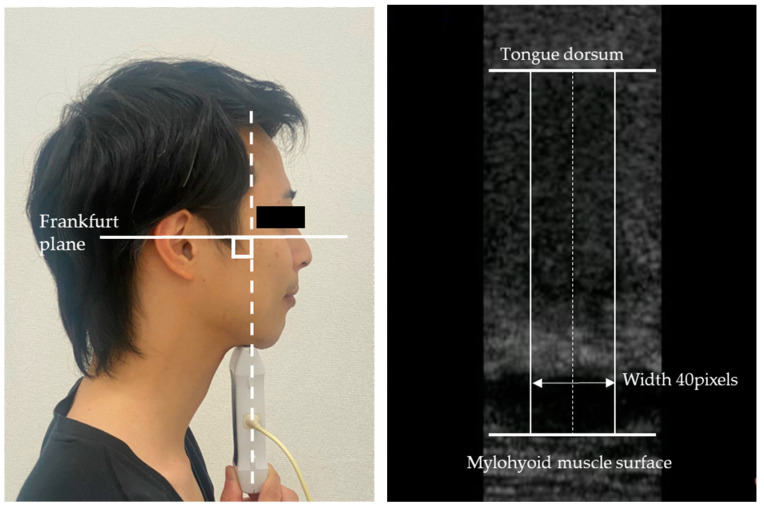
Echo intensity measurement of tongue using ultrasonography. Probe and head position (left), and measurement of echo image (right).
